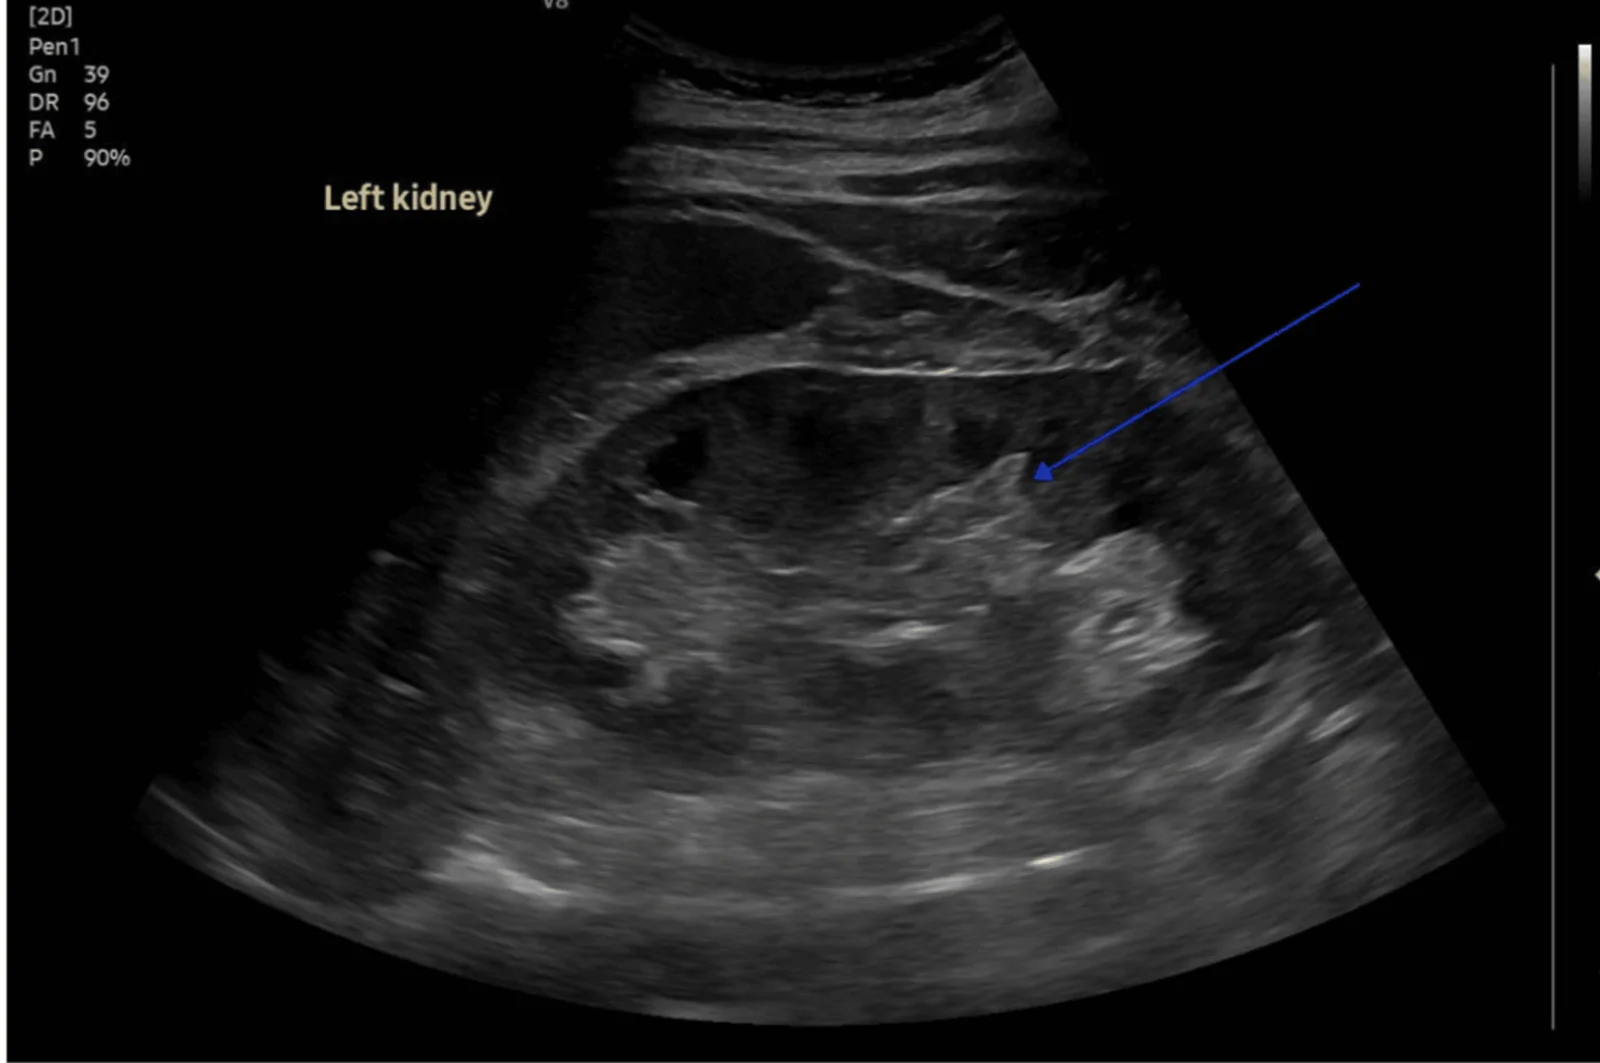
Ultrasound scan showing unobstructed left kidney with normal corticomedullary differentiation (blue arrow)
